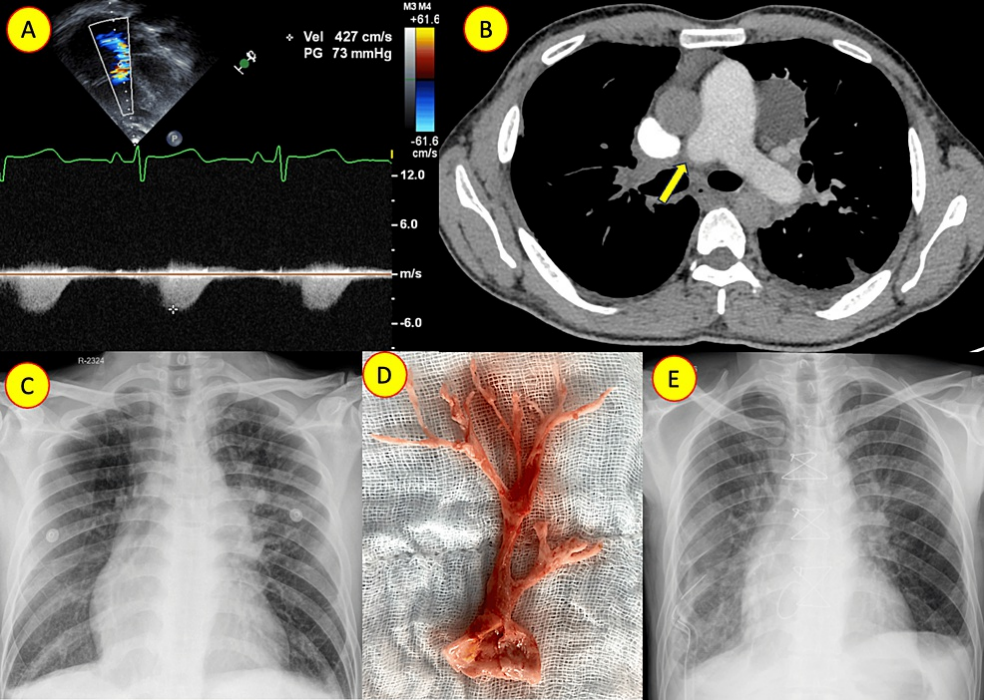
Pulmonary artery thromboembolism A: Continuous-wave Doppler revealing severe elevation of right ventricular systolic pressure, determined by the tricuspid regurgitation jet. B: Computed tomography pulmonary angiography demonstrating complete occlusion of the right pulmonary artery (yellow arrow). C: Chest X-ray (anterior-posterior view) exhibiting signs of differential vascularity and reduced right lung blood flow. D: A large thrombus recovered after removal. E: Chest X-ray (anterior-posterior view) post-thrombus removal, showing restored right lung blood flow.
